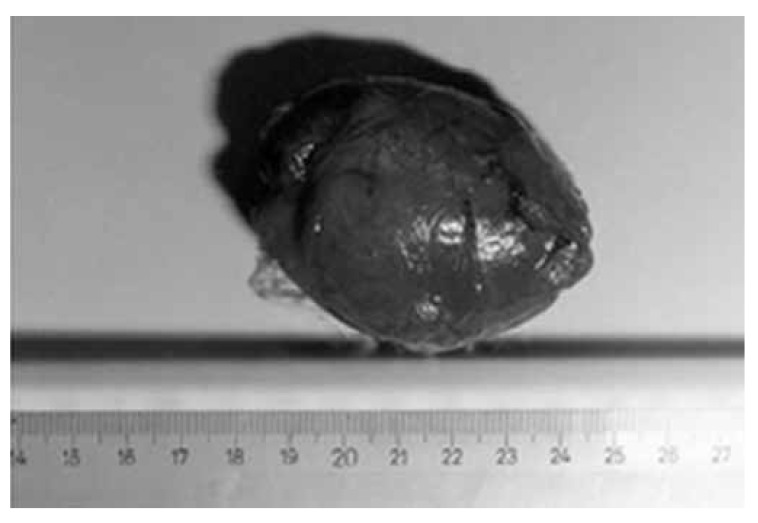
CT report: 4 × 5-cm hypo-dense lesion (myxoma) that caused a filling defect in the right atrium.
